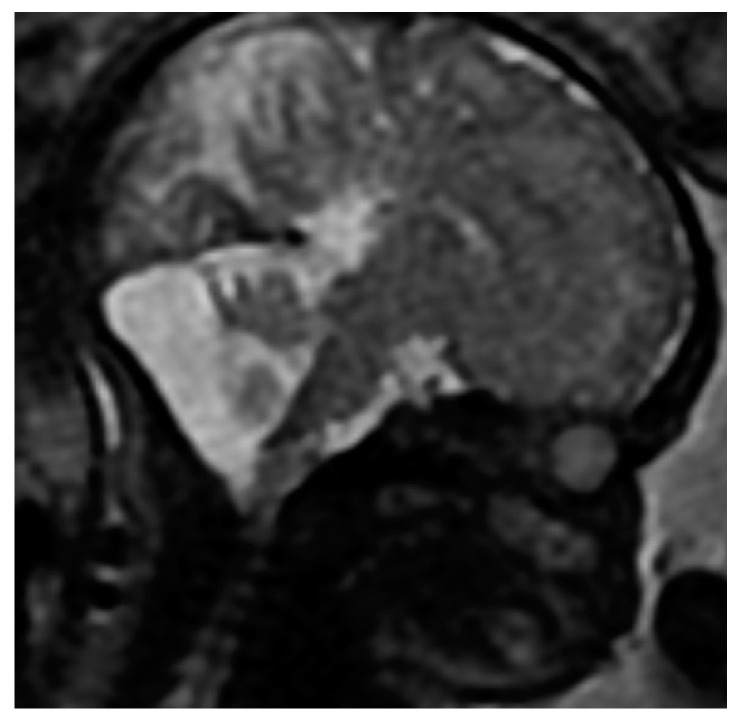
MRI picture of vermis dysgenesis.
